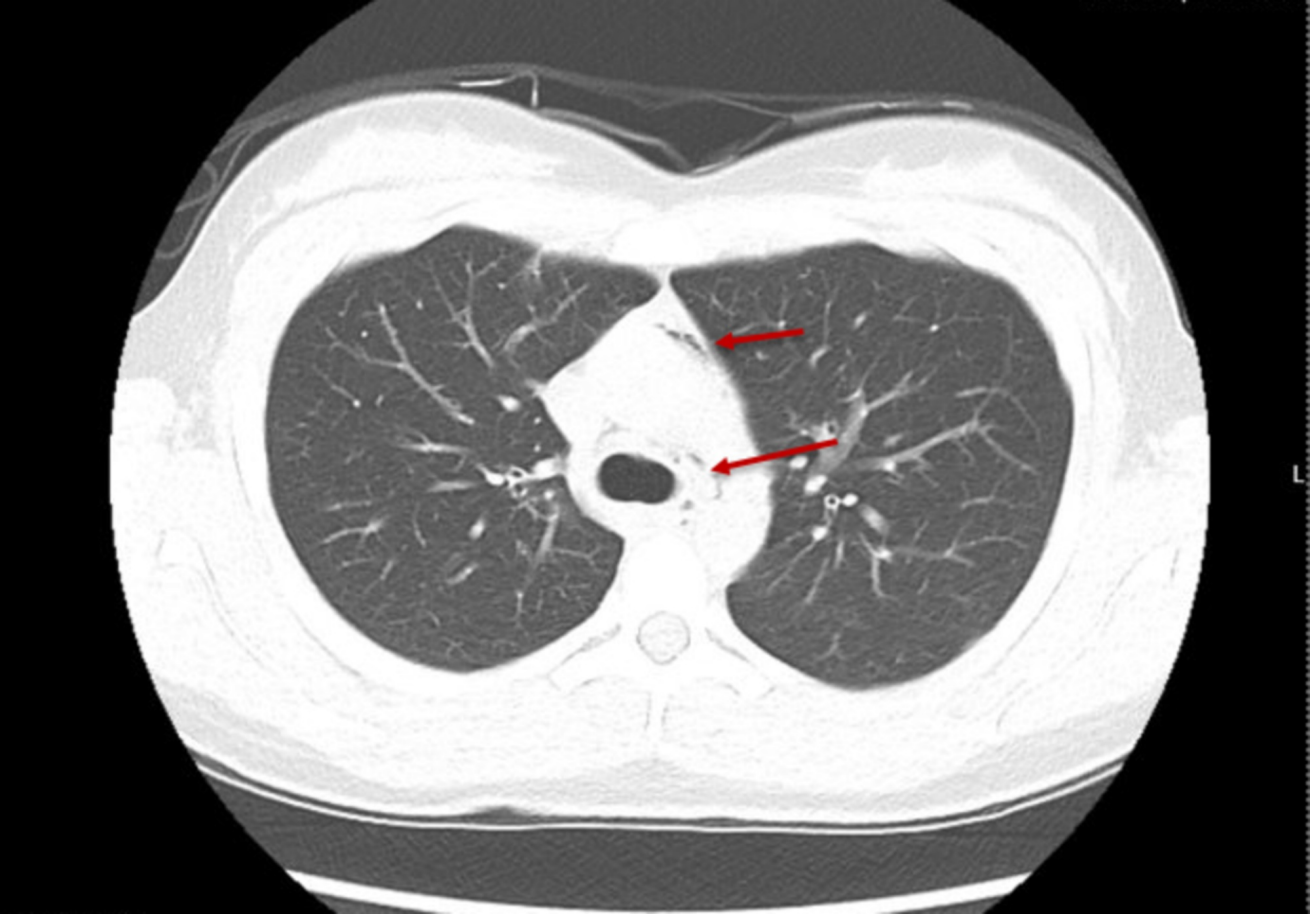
CT of the chest demonstrating the presence of air within the mediastinum, surrounding the pulmonary trunk (upper red arrow) and around the trachea (lower red arrow)
